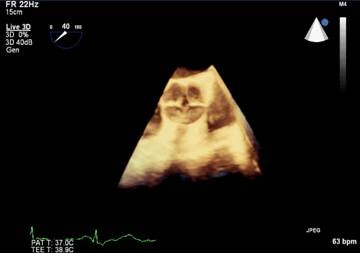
3-D transesophageal echocardiogram. The aortic valve opened and closed. It consiste of four cusps of equal size. This was clearer compared with those using 2-D transesophageal echocardiogram.
